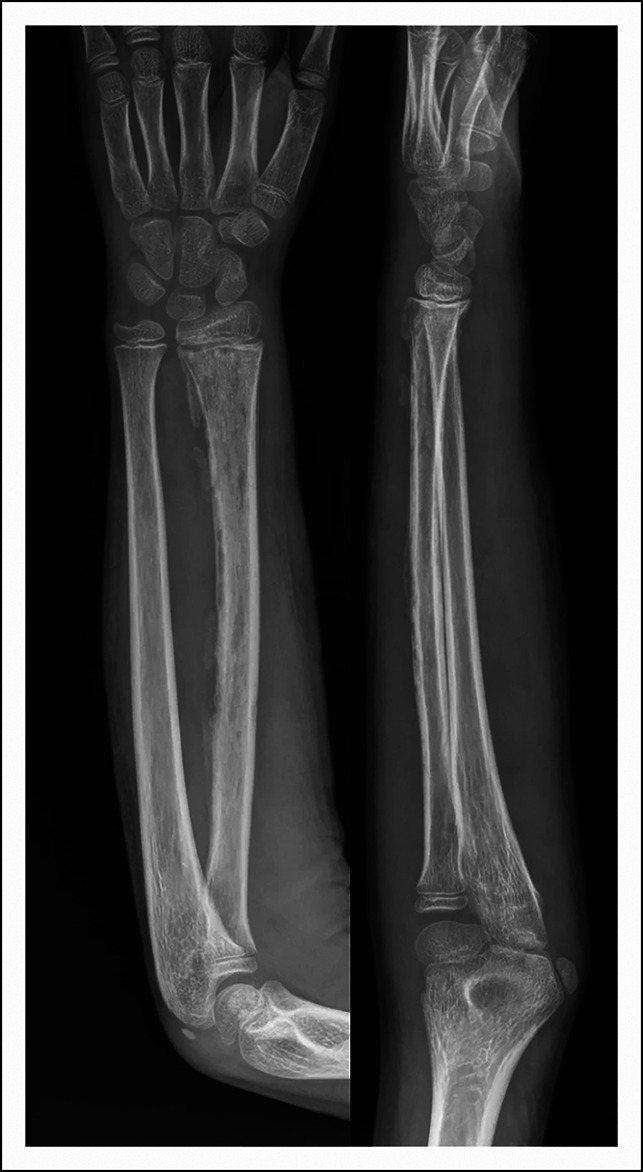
AP and lateral radiographs of the left forearm with regional osteopenia, periosteal reaction, and radial cortical erosion suggestive of osteomyelitis.
